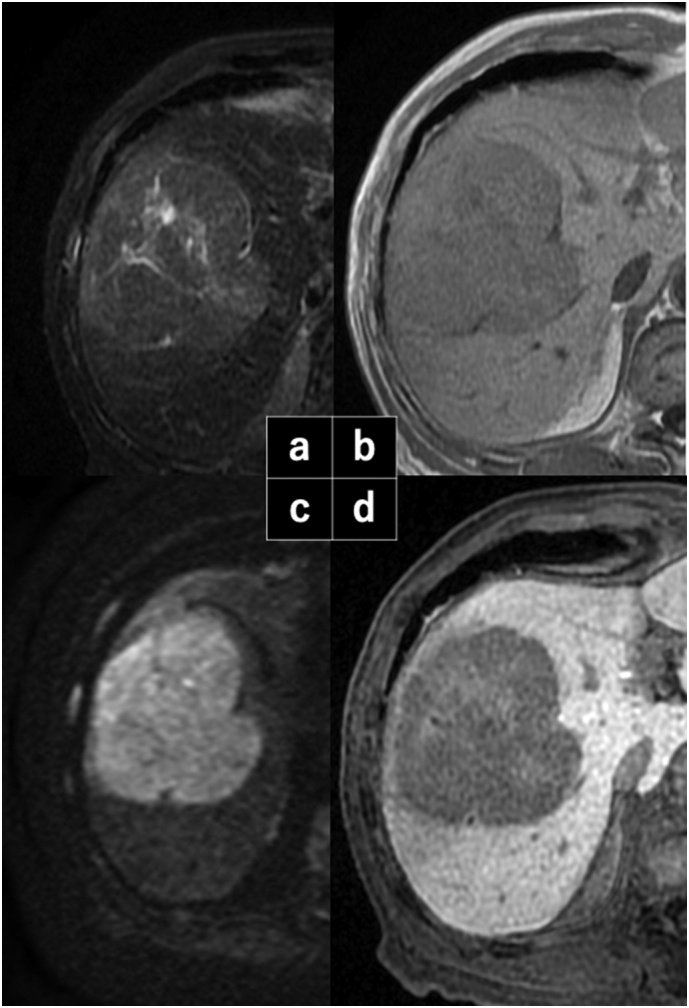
Hepatobiliary phase of gadolinium ethoxybenzyl-diethylenetriaminepentaacetic acid-enhanced magnetic resonance imaging. A 9-cm sized hepatocellular carcinoma in the right lobe of the liver was detected.
